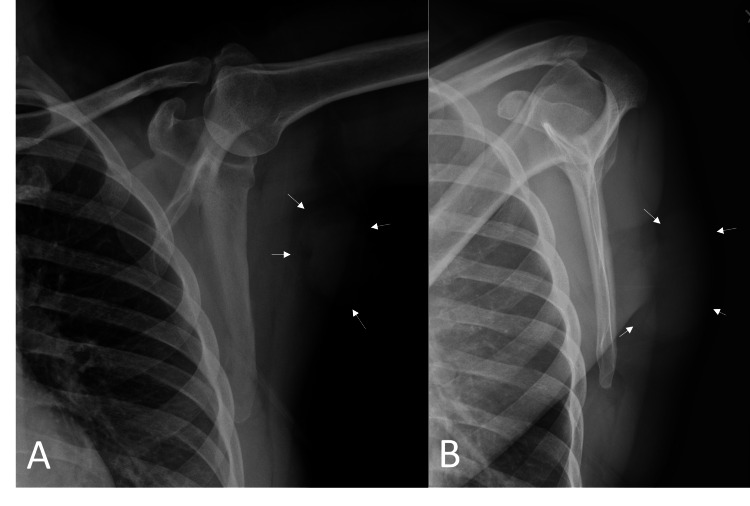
Plain radiography images (A, B) reveal a soft tissue mass (arrows) with no any bony abnormality.
